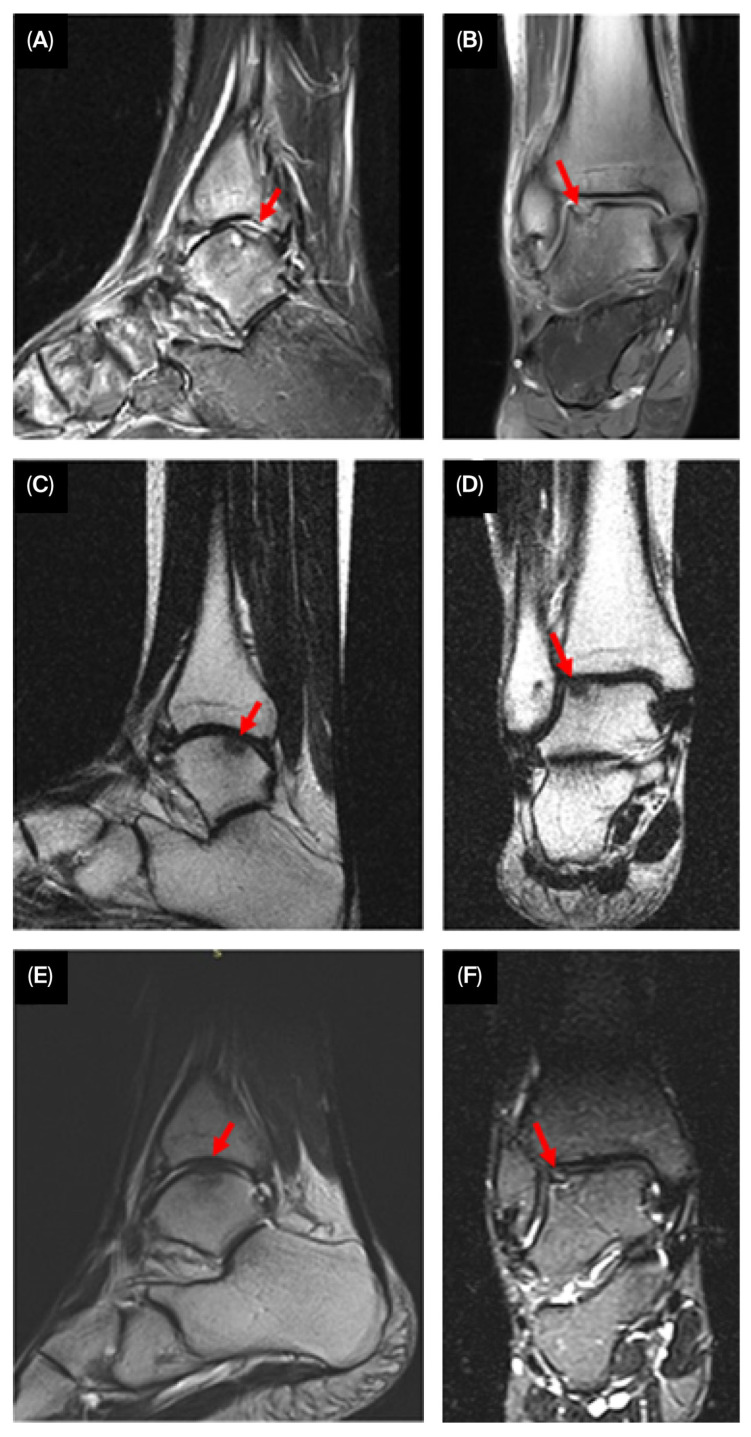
Serial MRI images showing progressive cartilage regeneration (arrowheads) and defect filling over 3 ((A)—sagittal, (B)—coronal), 6 ((C)—sagittal, (D)—coronal), and 12 months ((E)—sagittal, (F)—coronal).
